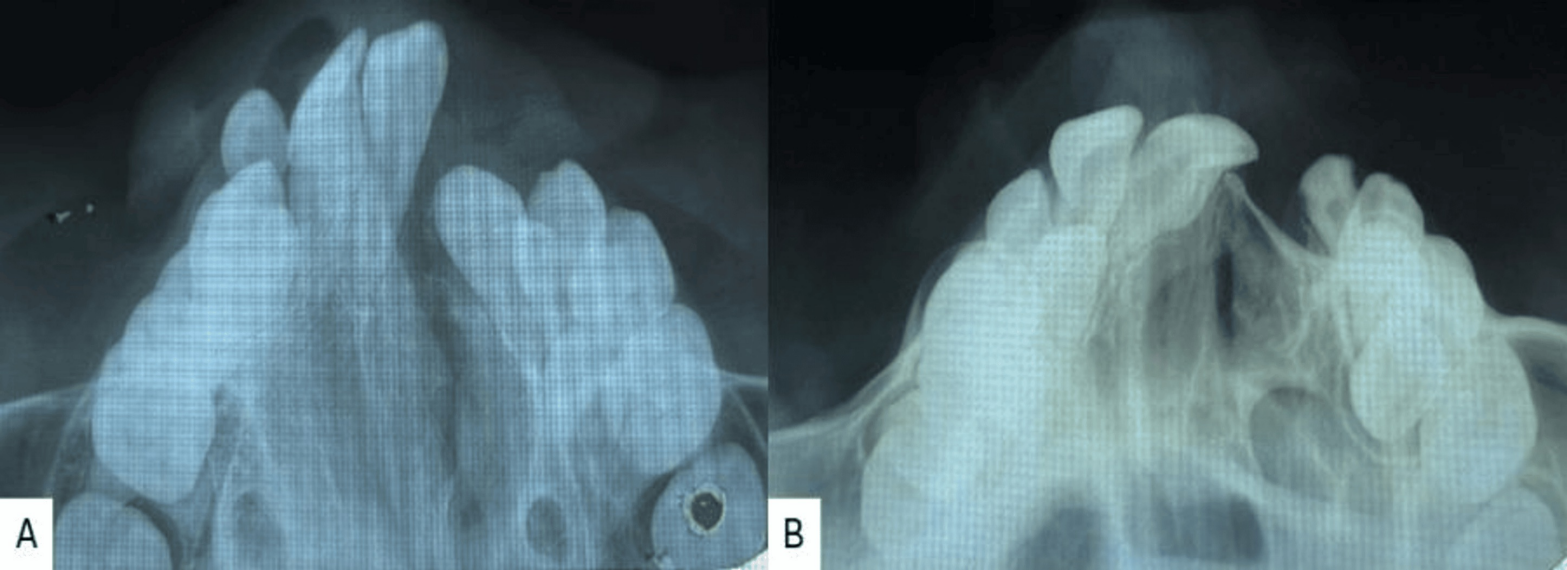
Radiographic comparison of the maxillary arch expansion A) Pre-treatment; B) post-expansion
